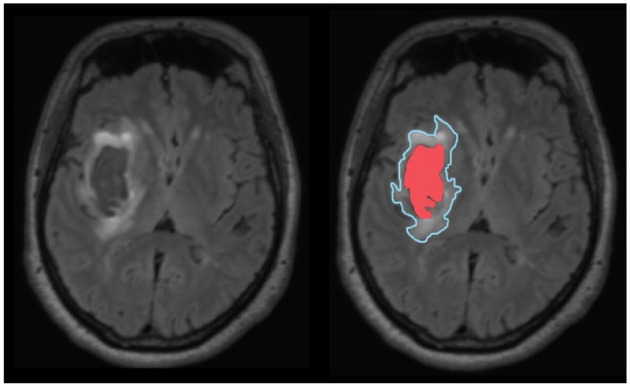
Example of the delineation of perihaematomal oedema (blue line) and the intracerebral haematoma (depicted in red) on a MRI T2-FLAIR scan.
